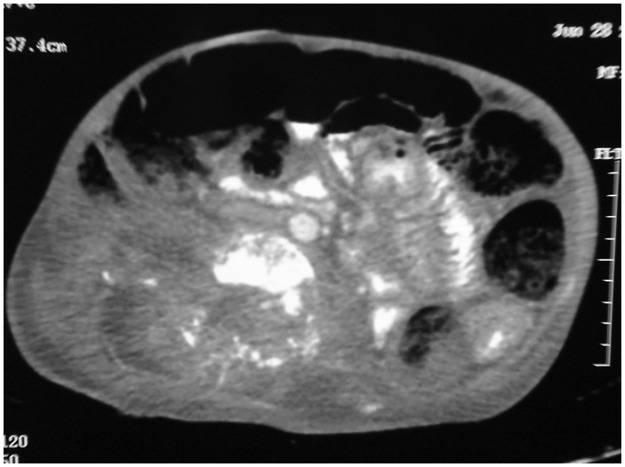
CT scan showing extensive cystic lesions and massive destruction of the lumber vertebrae, sacrum, and left iliac bone associated with paravertebral soft tissue mass extending to the psoas muscles.
